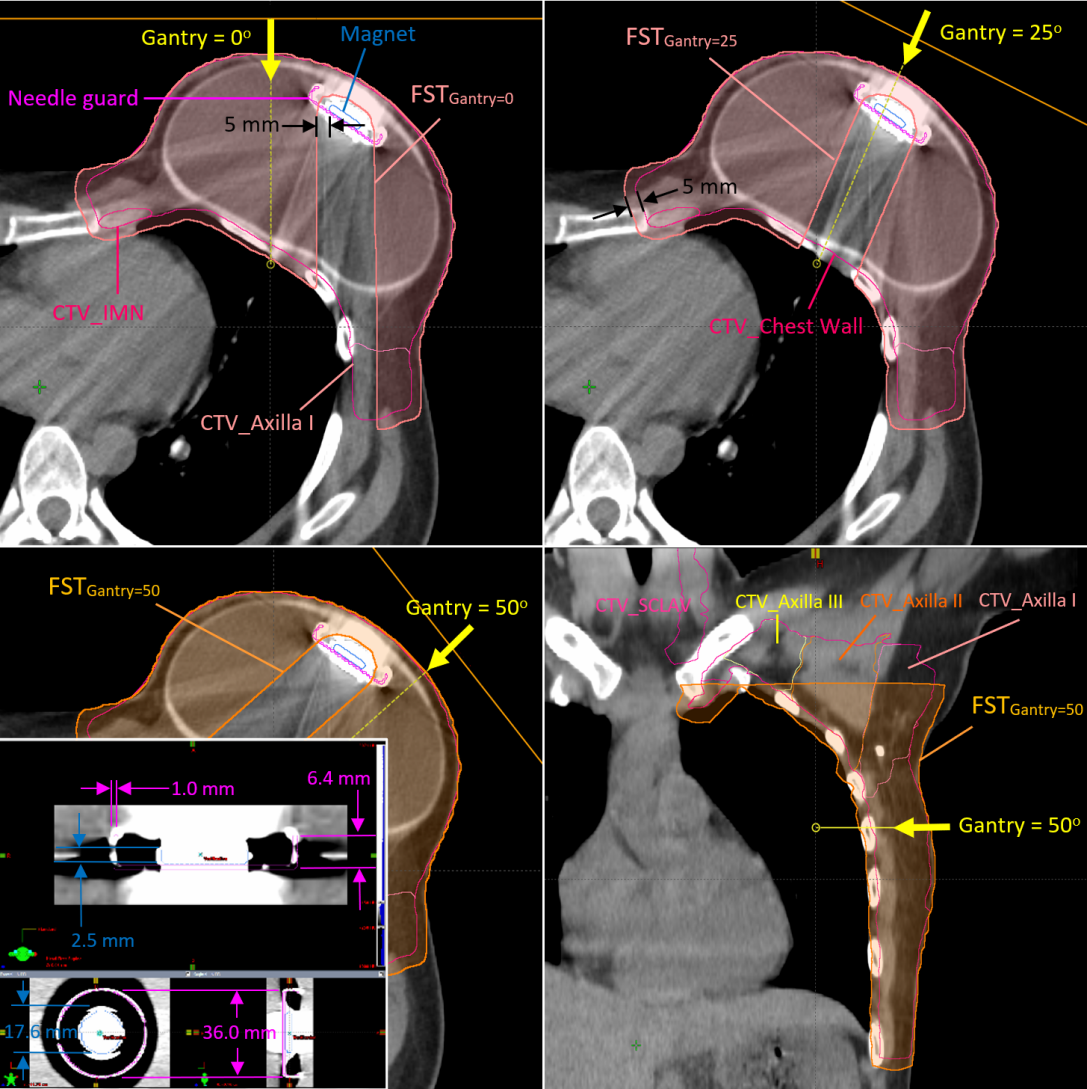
Clinical target volumes (CTVs) and the field-specific targets used for the fields at gantry angles of 0°, 25°, and 45° in Plan B The metallic port template in high-resolution CT image is also shown at the left-bottom corner of the figure.
